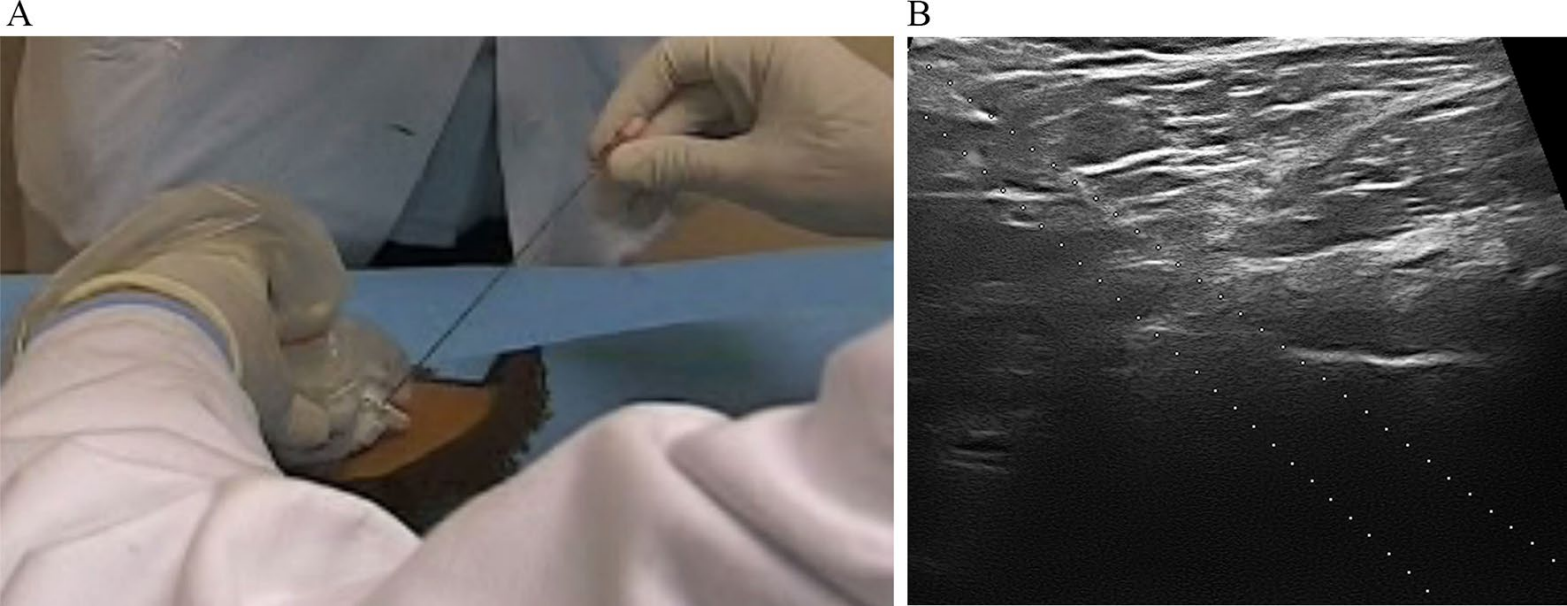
(A) Intra-articular injection performed by inserting a 18-gauge needle in the sterile guide with an antero-inferior approach; (B) intracapsular positioning of the needle was monitored in real time by direct US visualization of the needle on the screen of the ultrasound machine.
